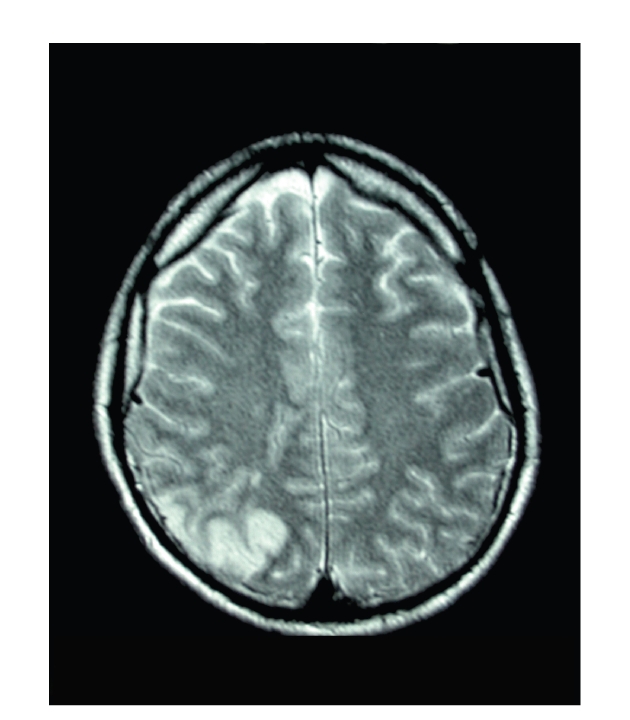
T2-weighted MRI showing bilateral hyperintense parieto-occipital lesions.
